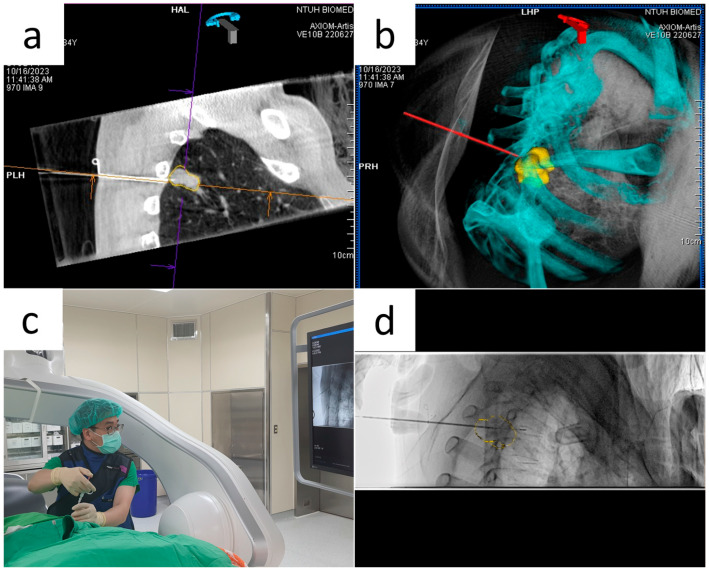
Needle insertion and confirmation during the biopsy procedure. (a) CBCT scan after initial needle insertion into the chest wall to ensure that the needle is on the planned route (iGuide toolbox used for lesion segmentation). (b) 3D reconstruction of the whole thorax to visualise the spatial relationships between the needle and the surrounding bony structures and enable rotation and rectification of deviations. The yellow mass shown is a 3-D segmentation of the target lesion. (c) The C-arm was turned to the progression view for further needle insertion under fluoroscopic guidance. (d) The needle is on target, as observed under the progression view on the monitor. The yellow contour indicates the lesion.
